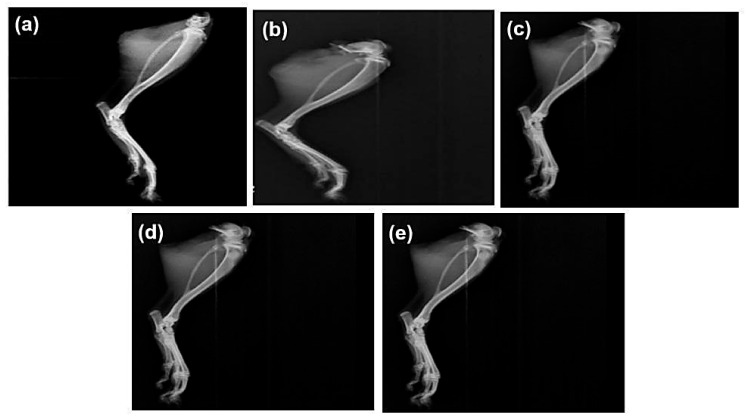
Effect of ALP and DSF on radiographs of the ankle joint in PO and MSU-induced GAR of (a) normal control, (b) disease control, (c) ALP (10 mg/kg), (d) DSF (50 mg/kg), and (e) ALP (10 mg/kg) + DSF (50 mg/kg).
